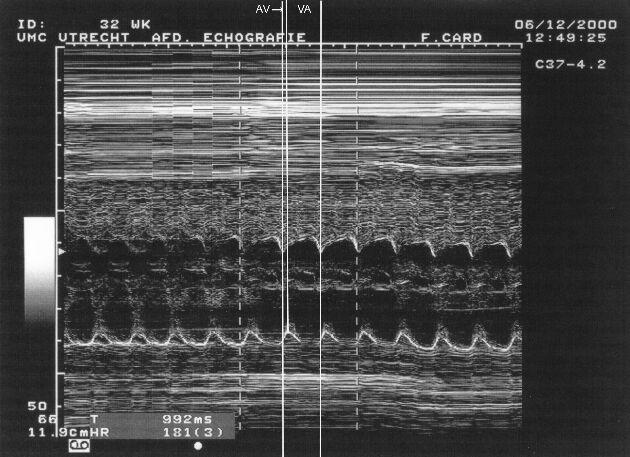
M-mode echocardiography of a SVT with a long VA interval
